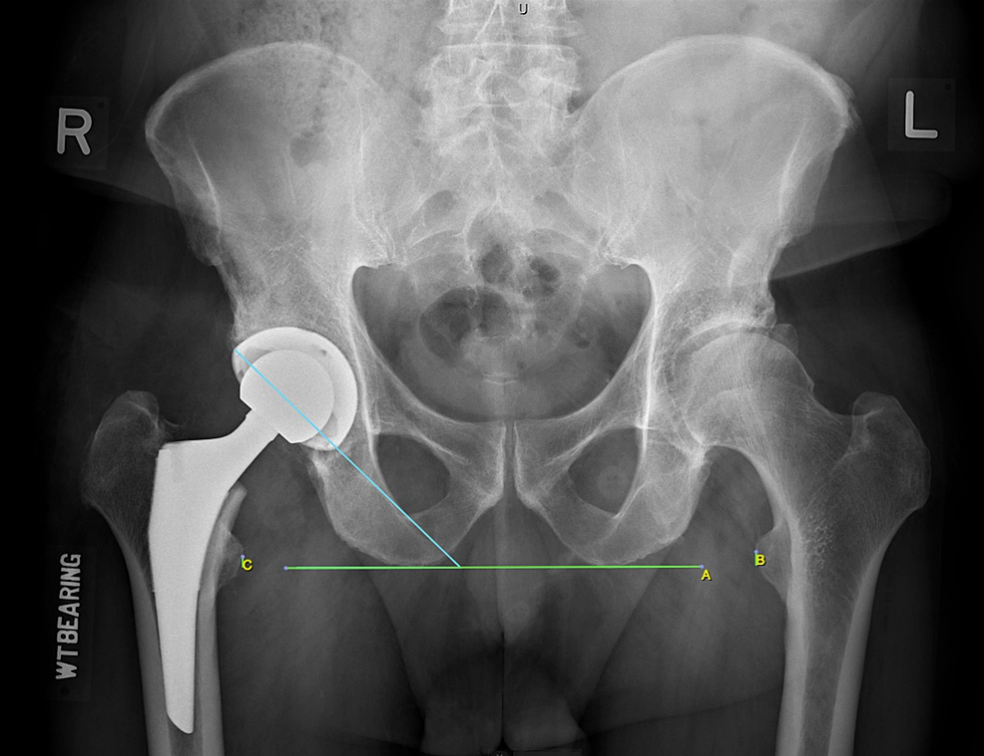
Secondary reference value. The secondary reference value was obtained from radiographic measurement using Osirix.
